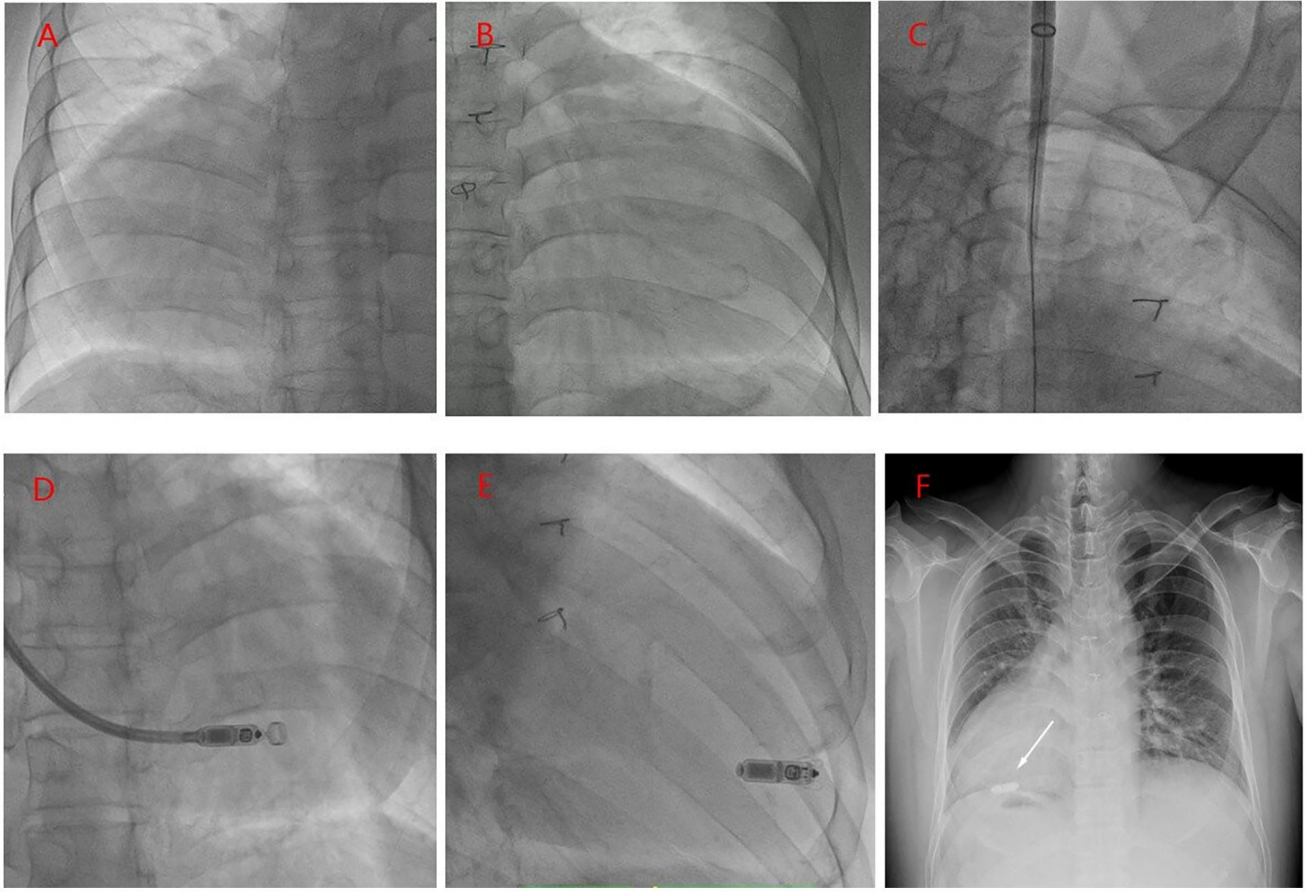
(A) RAO 30° fluoroscopy: Confirms a right-sided heart. (B) RAO 30° digital mirror view. (C) A 25 F delivery sheath was inserted via the left internal jugular vein, with its tip positioned in the right atrium. (D) A pre-loaded Micra AV controllable curved delivery system was advanced through the sheath, traversing the tricuspid valve and entering the anatomical right ventricle. (E) The leadless pacemaker was gradually deployed, with its distal tip anchored in the mid to lower ventricular septum. (F) Postoperative chest x-ray: The leadless pacemaker (white arrow) is correctly positioned against the mid-low ventricular septum without evidence of displacement.
